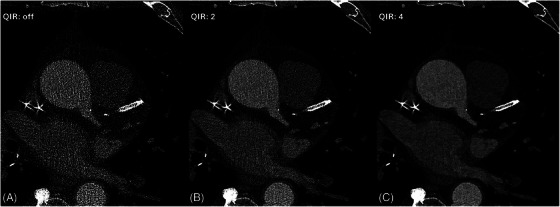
Axial coronary computed tomography angiography of an 80‐year‐old male using UHR PCD‐CT (0.2 mm slice thickness, sharp Bv64 kernel, window level 300, width 900) using different strengths of quantum iterative reconstruction (QIR). With no use of QIR images appear very noisy; stent assessment is not possible (A). With increasing strength of QIR (B,C) image impression becomes less noisy allowing for better assessment of smaller structures.
